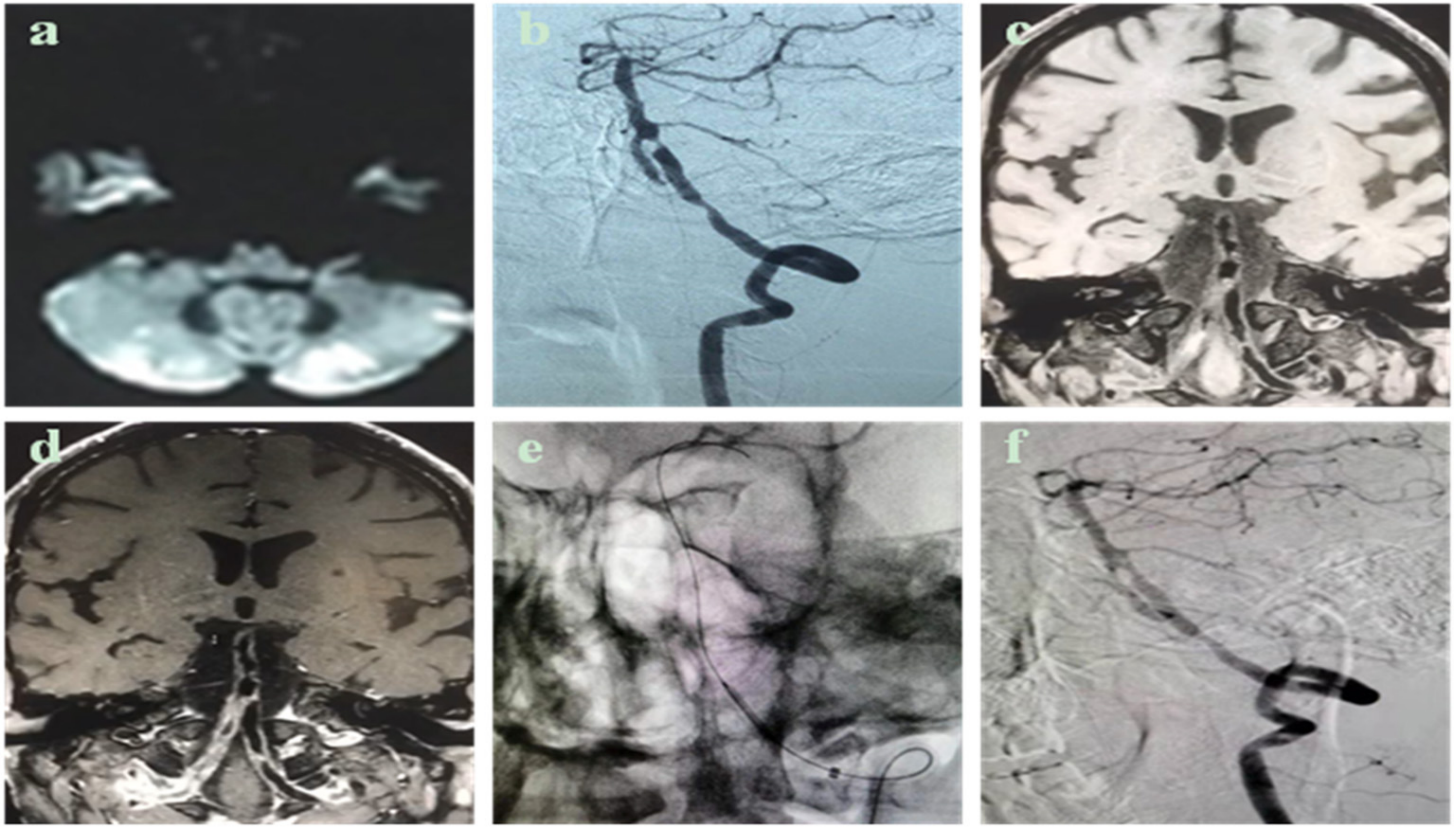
Case description: Male, 50 years old, admitted to the hospital for 1 month due to dizziness and walking instability, who was treated in an external hospital, and the head magnetic resonance imaging showed multiple acute cerebral infarctions in both cerebellar hemispheres. He has a history of hypertension and cerebral infarction and has received antiplatelet and lipid-lowering therapy. Have a history of smoking. Cerebral angiography shows occlusion of the medial segment (V4) of the right vertebral artery and severe tandem stenosis of the V4 segment of the left vertebral artery. After admission, high-resolution magnetic resonance imaging of the intracranial vascular wall was completed, suggesting that the V4 segment of the left vertebral artery was a narrowing caused by atherosclerotic plaque. (a) Cranial MRI DWI sequence shows multiple points and flaky hyperinflation of the cerebellar hemisphere on both sides; (b) cerebral angiography sees occlusion of the V4 segment of the right vertebral artery, 70% of the proximal stenosis of the V4 segment of the left vertebral artery, and 90% of the proximal fusion segment of the distal, proximal fusion segment; (c,d) high-resolution MRI vascular wall imaging shows an eccentricity of the V4 segment of the left vertebral artery, focal thickening, local bleeding in the plaque, plaque strengthening; (e,f) The left vertebral artery V4 segment tandem stenosis lesion is pre-dilated sequentially with a balloon of 2.0 mm × 15 mm. The proximal stenosis was placed with a 3 mm × 13 mm spherical expansion stent, and the distal stenosis was placed with a 2.5 mm × 10 mm spherical expansion stent, and the post-operative angiography showed that the left vertebral artery V4 segment tandem stenosis improved, and the residual stenosis ≤30%.
